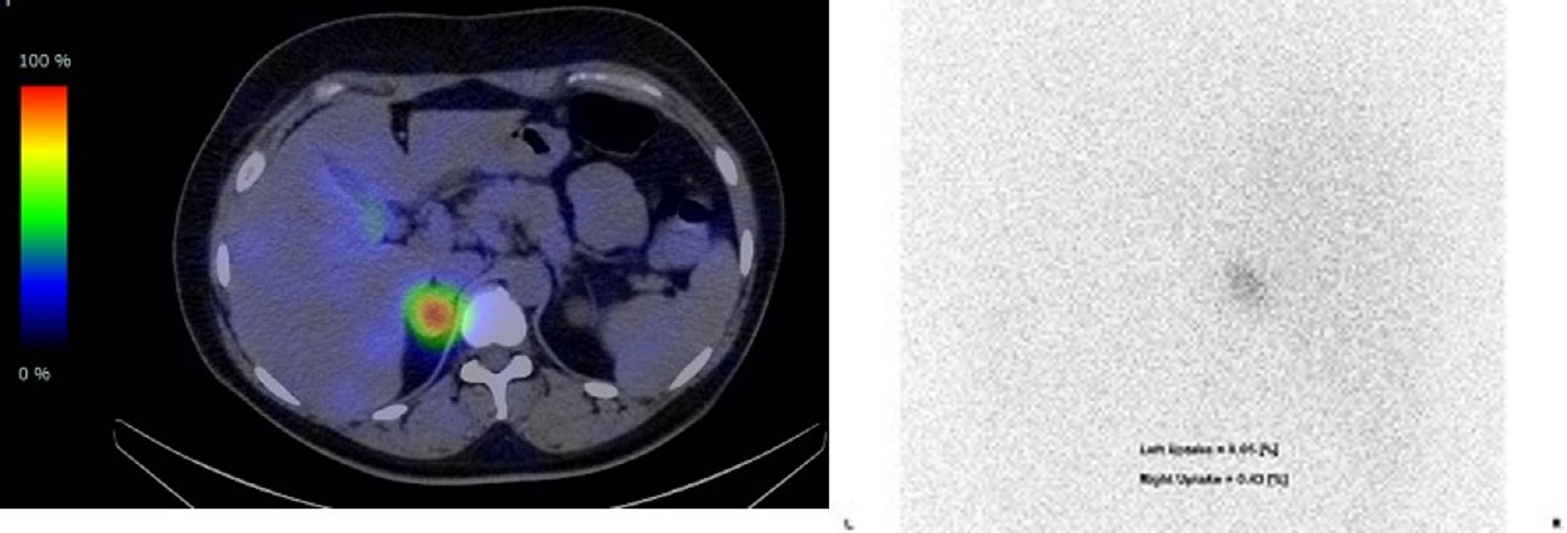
Adosterol adrenal scintigraphy on 2 months postpartum (There is an uptake only on the tumor side.)
